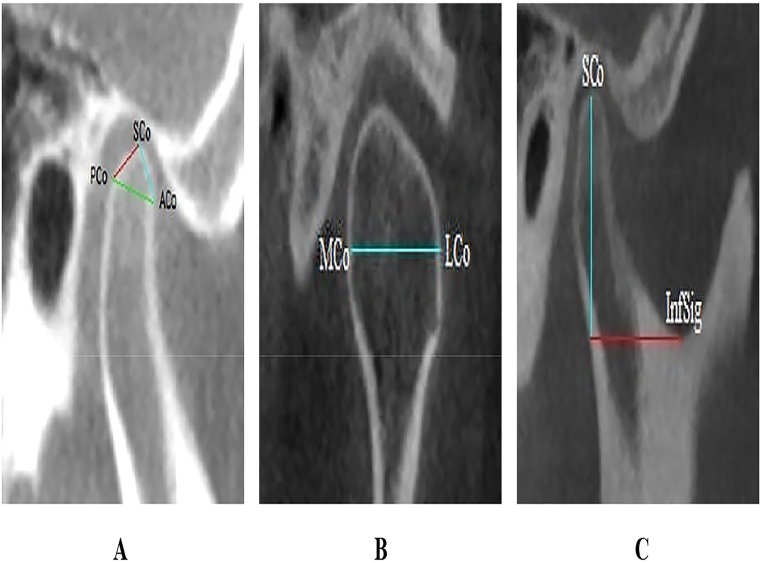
Condyle size measurements. CBCT images show the methods of measuring the condyle size. 1A. Sagittal view showing condylar length measurement. 1B. Coronal view showing condylar width measurement. Fig. 1C. Sagittal view showing condylar height measurement.
